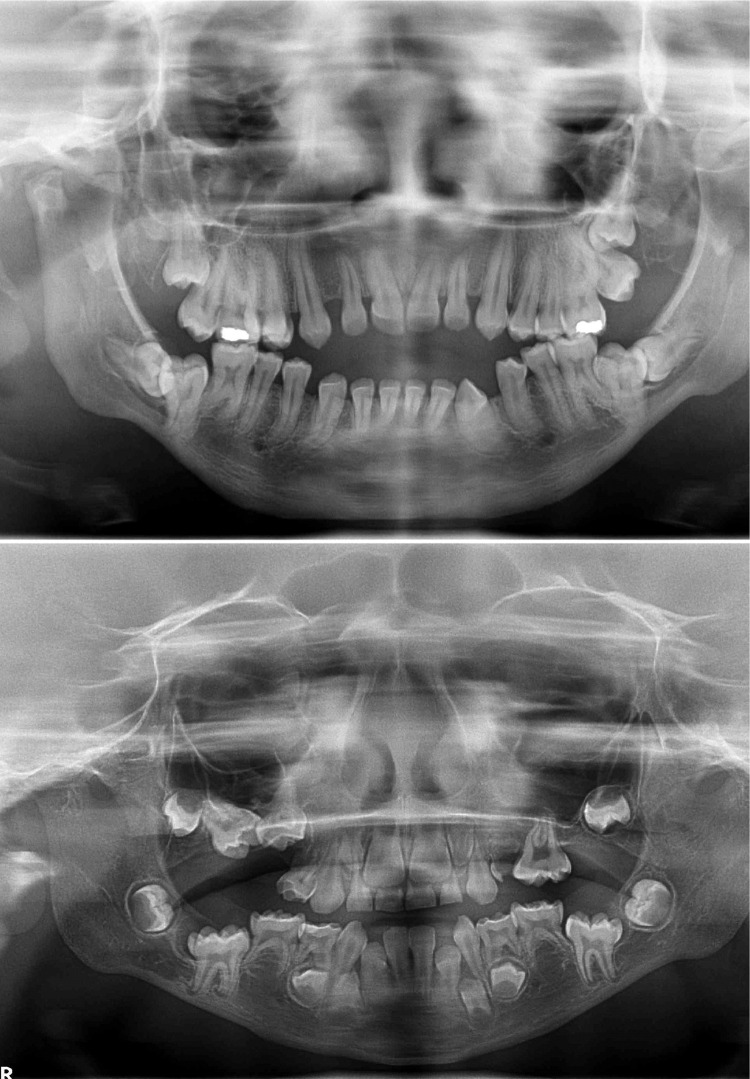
Panoramic radiographs of individuals with MPS type VI, aged 18 years old (A) and MPS type I, aged 6 years old (B), showing taurodontism, root dilaceration, tooth agenesis, and tooth impaction
